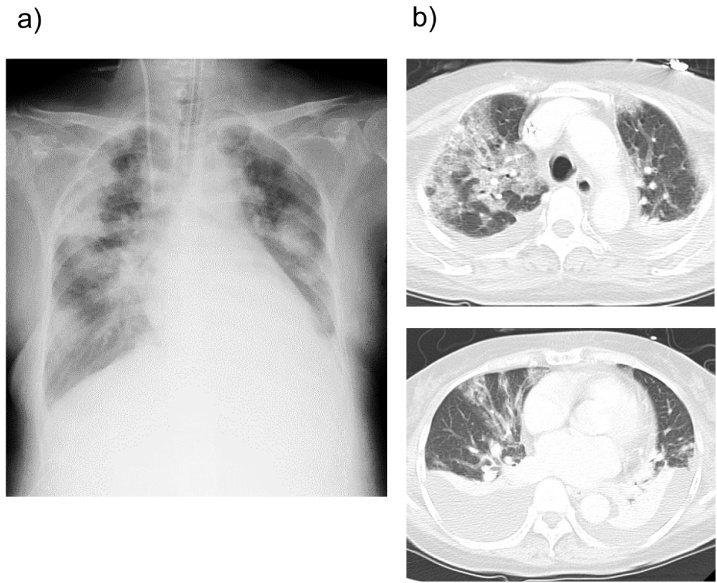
a) Radiograph showing an expanded cardiothoracic ratio (CTR) and bilateral field opacities in a butterfly distribution on POD 4. b) Chest CT showing diffuse ground-glass appearance and pleural effusion in bilateral lung fields.
